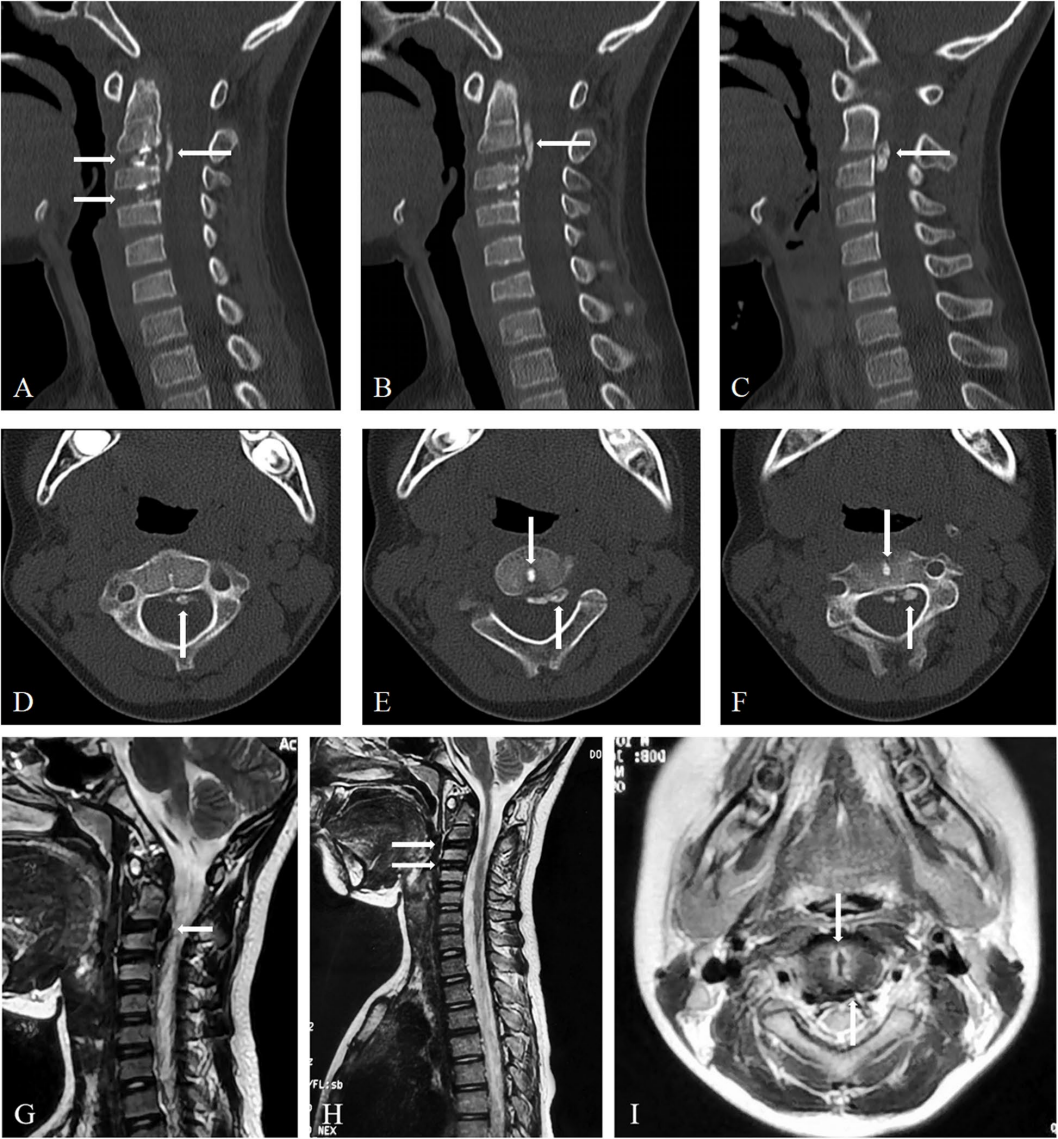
A-F CT images of cervical vertebra depict intervertebral disc calcification at the C2/3 and C3/4 levels, with protrusion of OPLL at posterior edge of the C2 vertebral body and the C2/3 intervertebral disc level, encroaching into the spinal canal; G-I MRI scans of cervical spine demonstrate a reduction in signal intensity in T2WI for the C2/3 and C3/4 intervertebral discs, as well as the posterior longitudinal ligament of the C2 vertebral body. In the transverse plane, the PLL at the C2/3 level exhibits central-left herniation, resulting in slight compression of the dural sac
